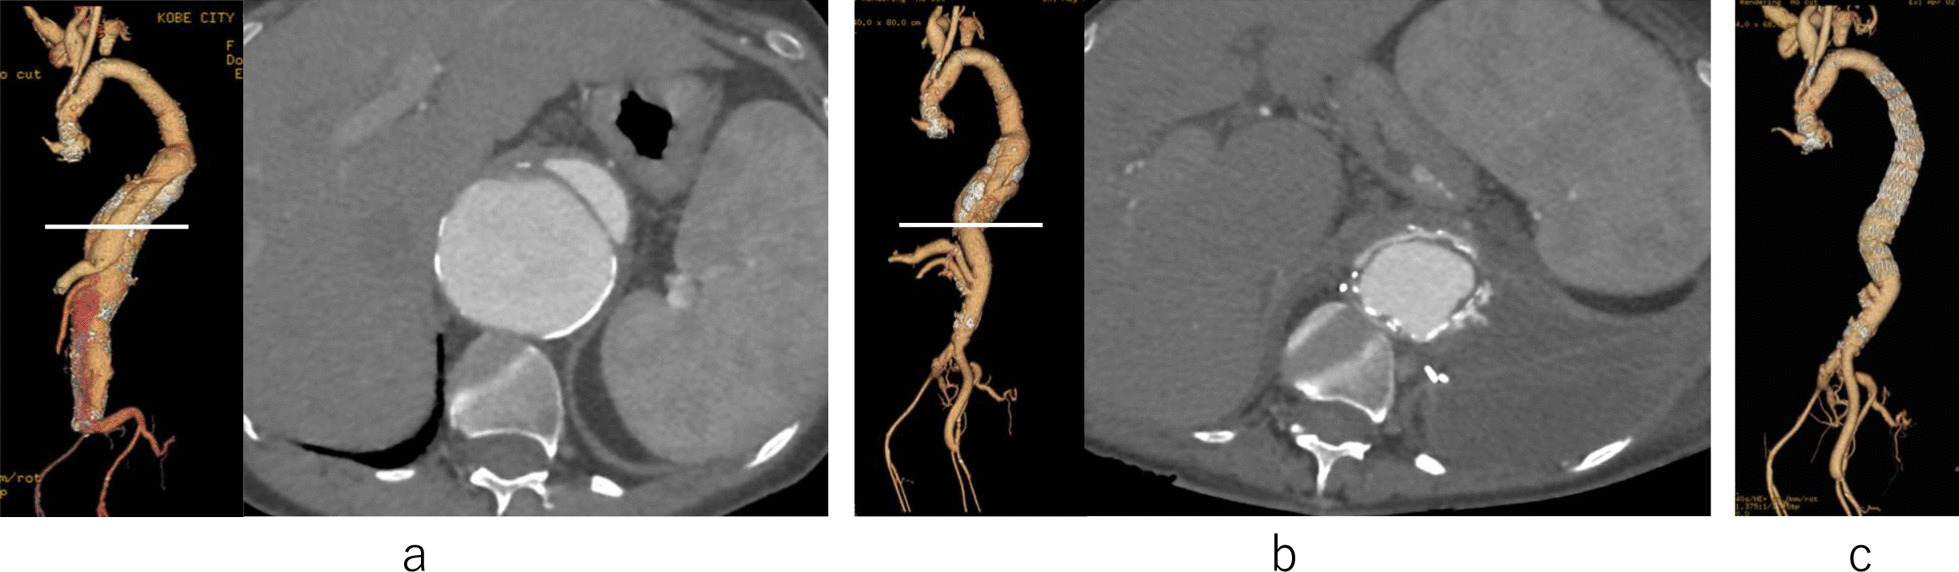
Three-dimensional computed tomography angiography (3D-CTA) images of case 1. a An axial cross-sectional image demonstrating the proximal anastomosis site (white line) in thoracoabdominal aortic replacement; b an axial cross-sectional image demonstrating the proximal anastomosis site (white line) after the primary open surgery. The peripheral anastomosis is the left external iliac artery; c the transabdominal aorta after hybrid repair
